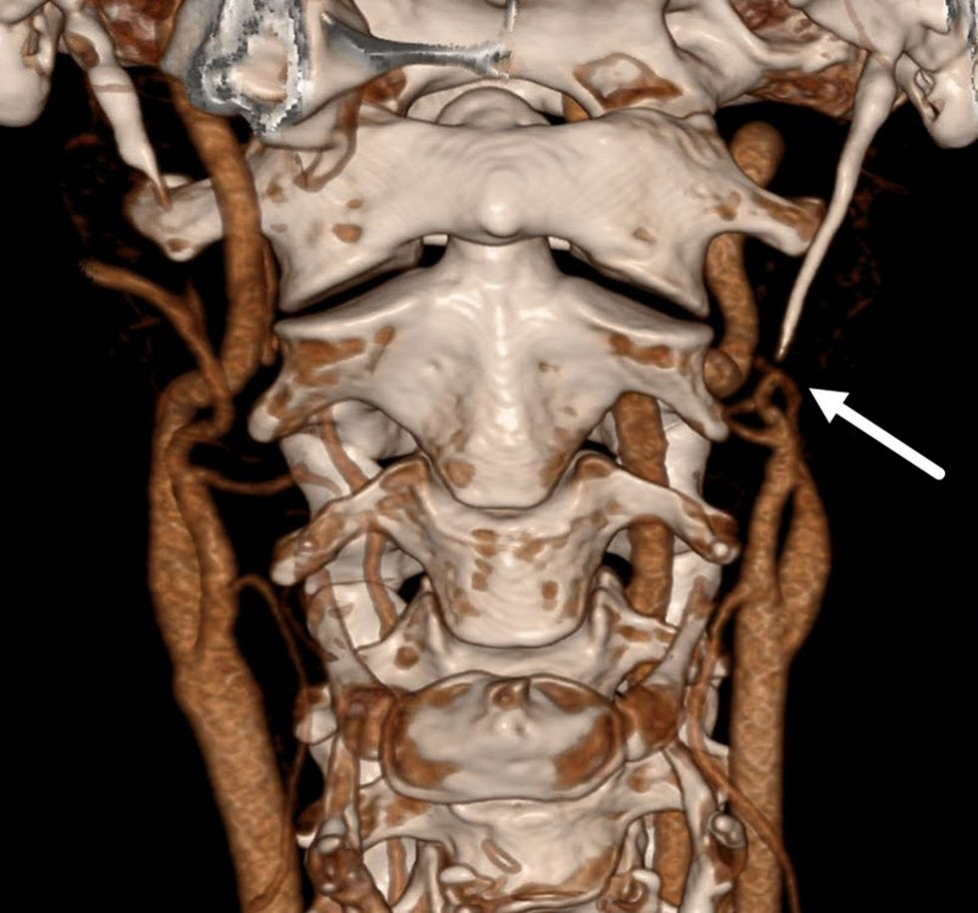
3D reconstruction of the CT-angiography shows a complete occlusion of the left ICA starting from the point of contact with the tip of the SP (white arrow). CT– computed tomography, ICA– internal carotid artery, SP– styloid process
